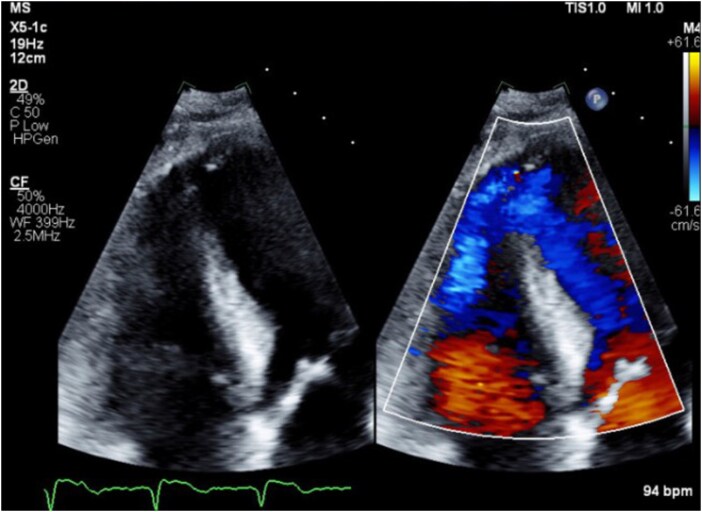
Transthoracic echocardiography demonstrating apical ventricular septal rupture (VSR). Apical four-chamber view with colour Doppler imaging shows a defect in the apical interventricular septum with continuous left-to-right shunting.
